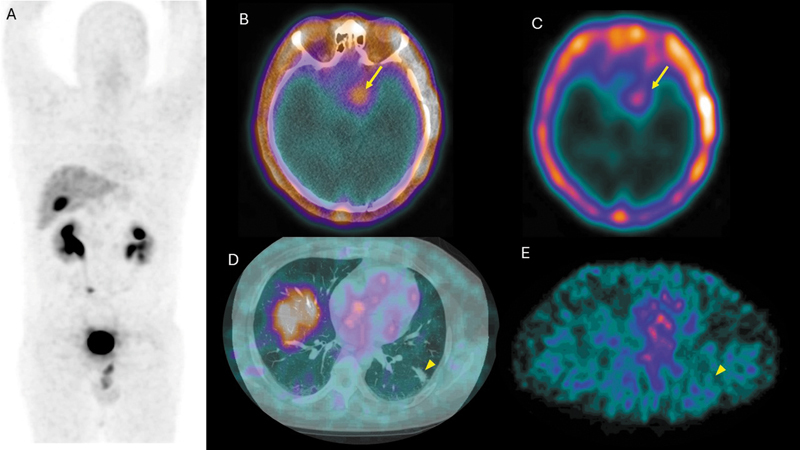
The figures show 99mTc-iFAP SPECT/CT images: (
